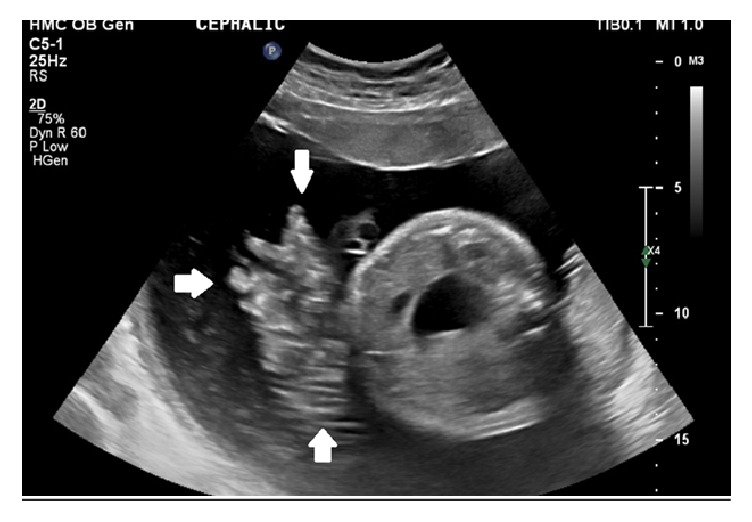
Ultrasound image of fetal blood swirling (arrows) into the amniotic fluid from the umbilical cord adjacent to a transverse view of the fetal abdomen/pelvis.
